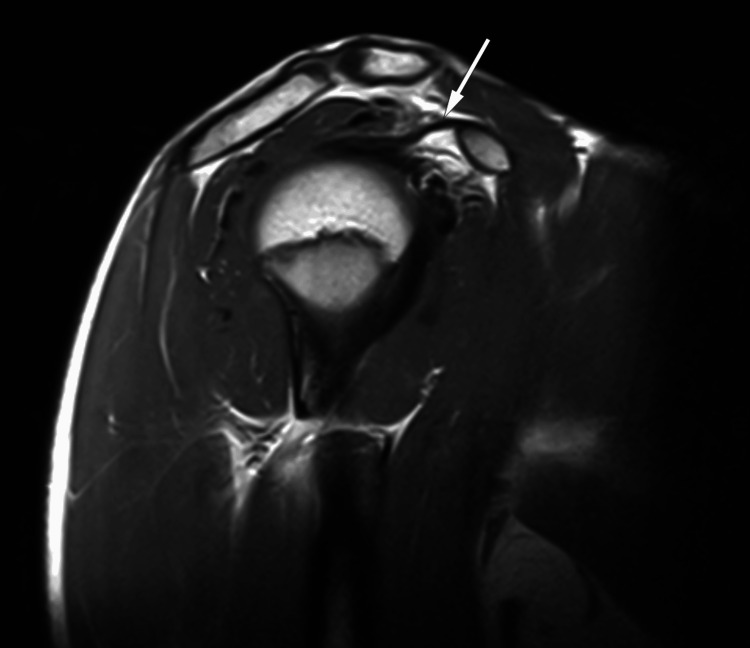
Sagittal proton density-weighted MRI of the right shoulder Demonstrating preserved integrity of the coracohumeral ligament, with normal appearance of the rotator interval and no evidence of bicipital pulley system injury
